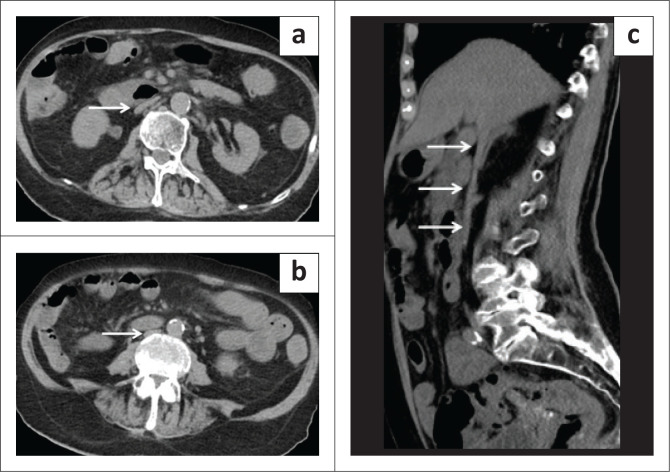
An 83-year-old female with gastrointestinal bleeding, haemorrhagic shock and renal dysfunction. Unfortunately, the patient expired within a few hours of imaging. The CT axial and sagittal images show a markedly flattened inferior vena cava (white thin arrows).
